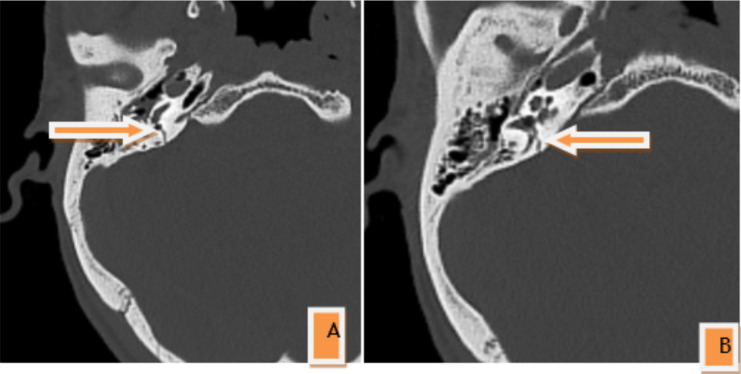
Axial non contrast bone window CT scan of a 35 years old male patient showing Right petrous temporal bone, otic capsule violating transverse fracture with involvement of the right ICA(A) and jugular fossa walls (B)
